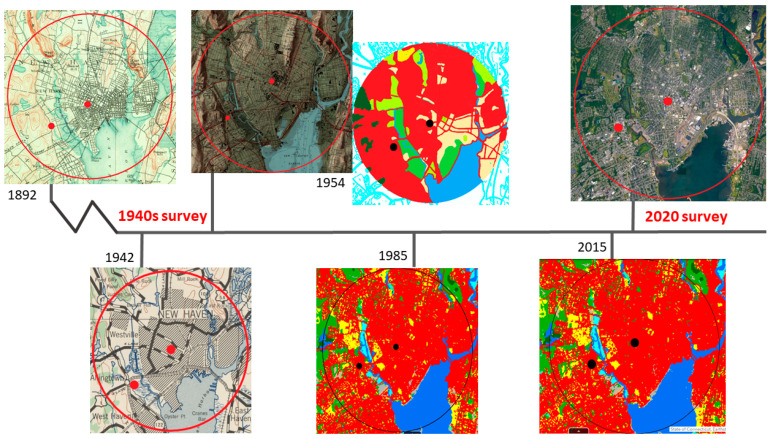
Topography and land cover of New Haven, CT, from 1892–2015. Source: 1892 [51]; 1942 [52]; 1954 [53]; 1954 land cover [47]; 1985 and 2015 land cover [50]; 2020 [54].
